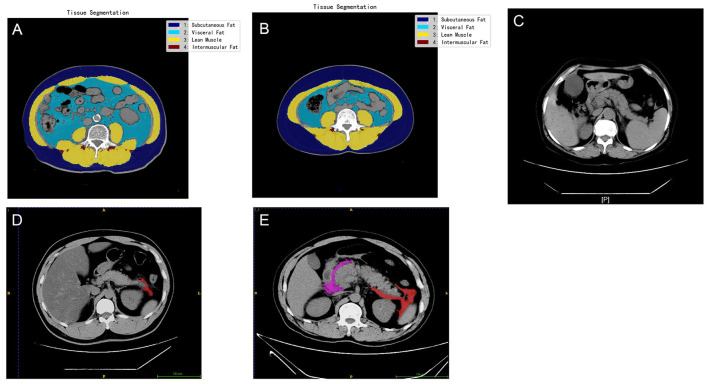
The non-enhanced computed tomography (CT) imaging for quantification of visceral fat and retroperitoneal inflammation. The inflammatory area is displayed in dark colors. (A) A 32-year-old male patient exhibited a visceral fat area of 116.389 cm^2^ and a subcutaneous fat area of 274.688 cm^2^, resulting in a visceral to subcutaneous fat ratio of 0.424. (B) A 58-year-old female patient had a visceral fat area of 64.035 cm^2^ and a subcutaneous fat area of 163.047 cm^2^, yielding a visceral to subcutaneous fat ratio of 0.393. (C) A patient without retroperitoneal inflammation. (D) A patient with left-sided retroperitoneal inflammation. (E) A patient with bilateral retroperitoneal inflammation.
